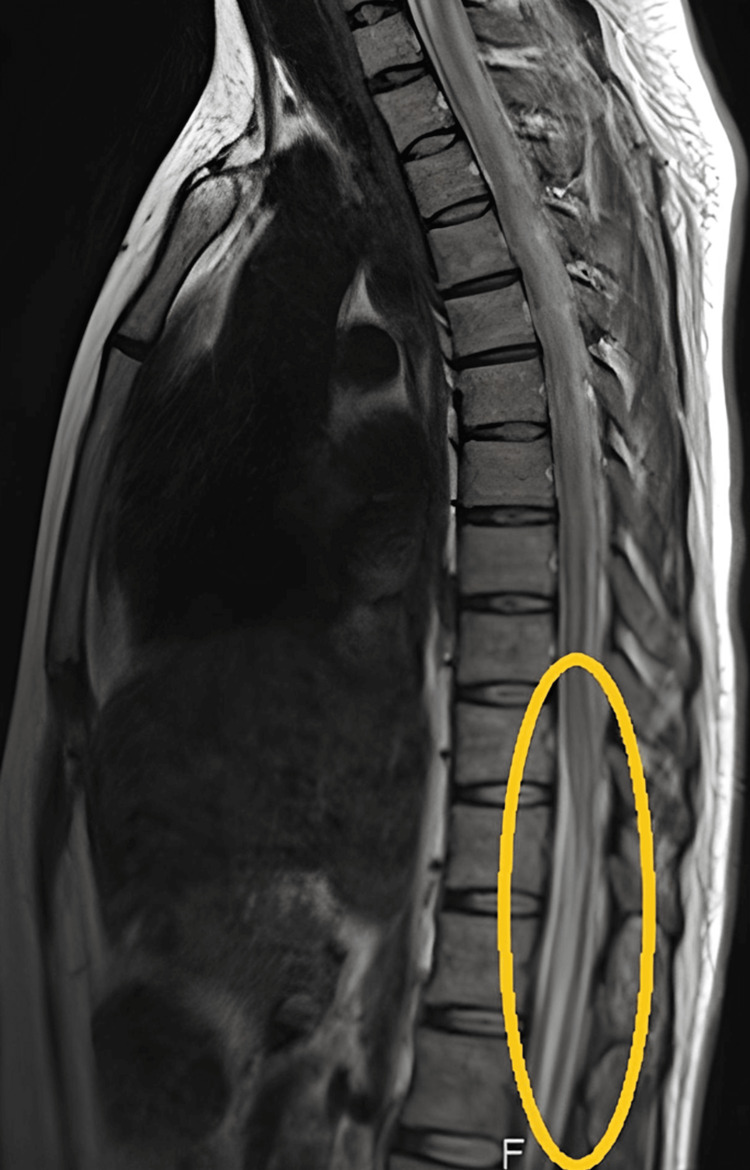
MRI spine showing transverse myelitis with mild disc desiccation at L5-SI level
